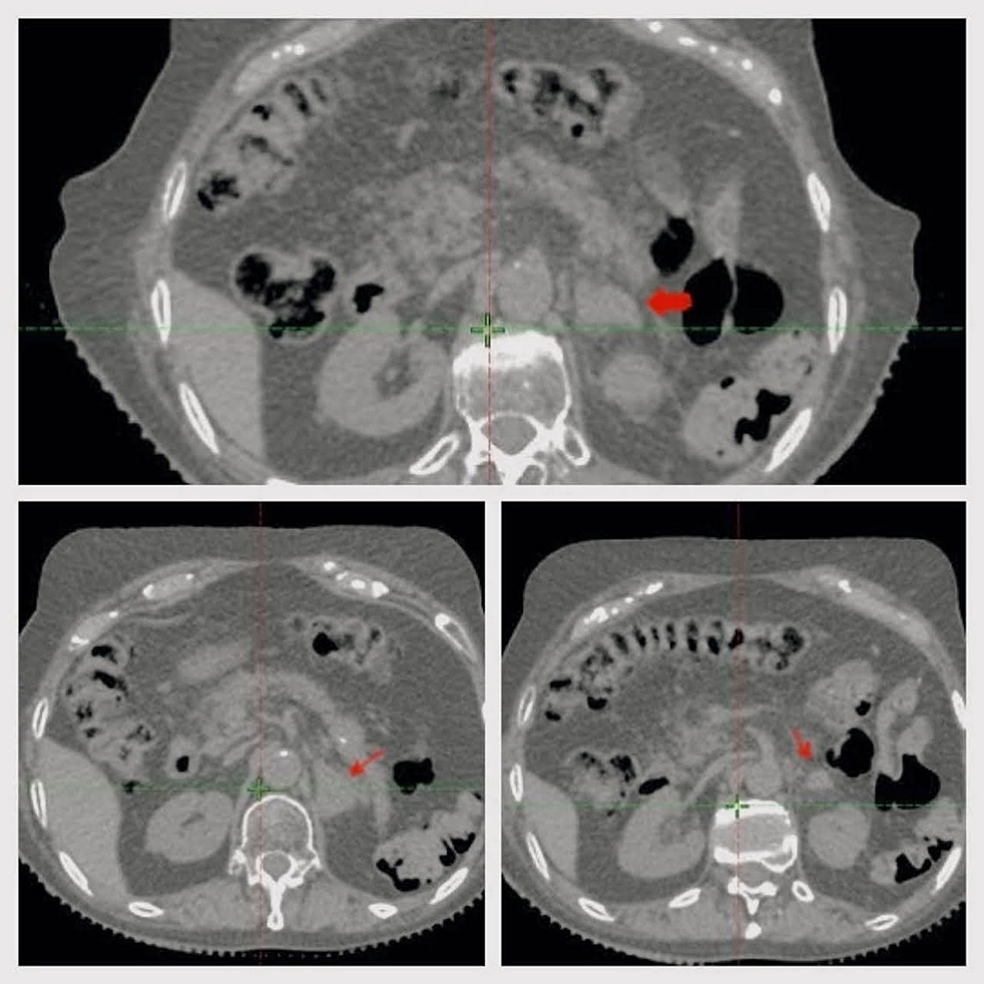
Pre-treatment abdominal CT A mass of soft tissue density can be identified in the left adrenal region, which extends even in front of the upper pole of the left kidney (arrow). No more pathological alterations are identified in the image.
